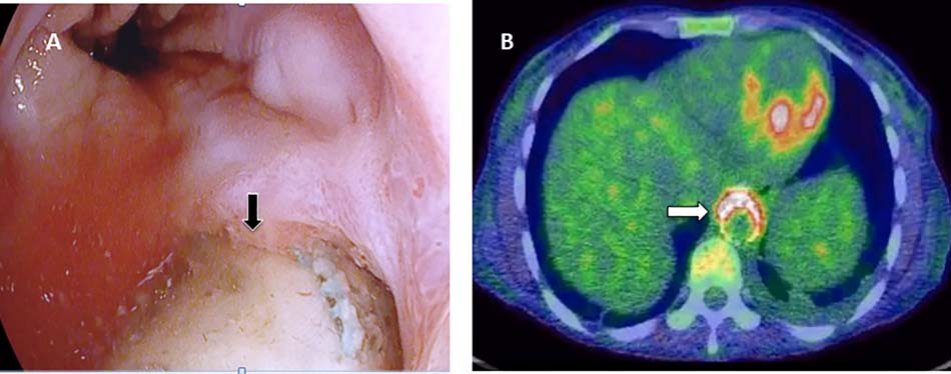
Esophagogastroduodenoscopy revealed thoracic endovascular aortic stent can be seen in the distal esophagus. (B) PET scan showing increase FDG activity around the distal aortic stent.
